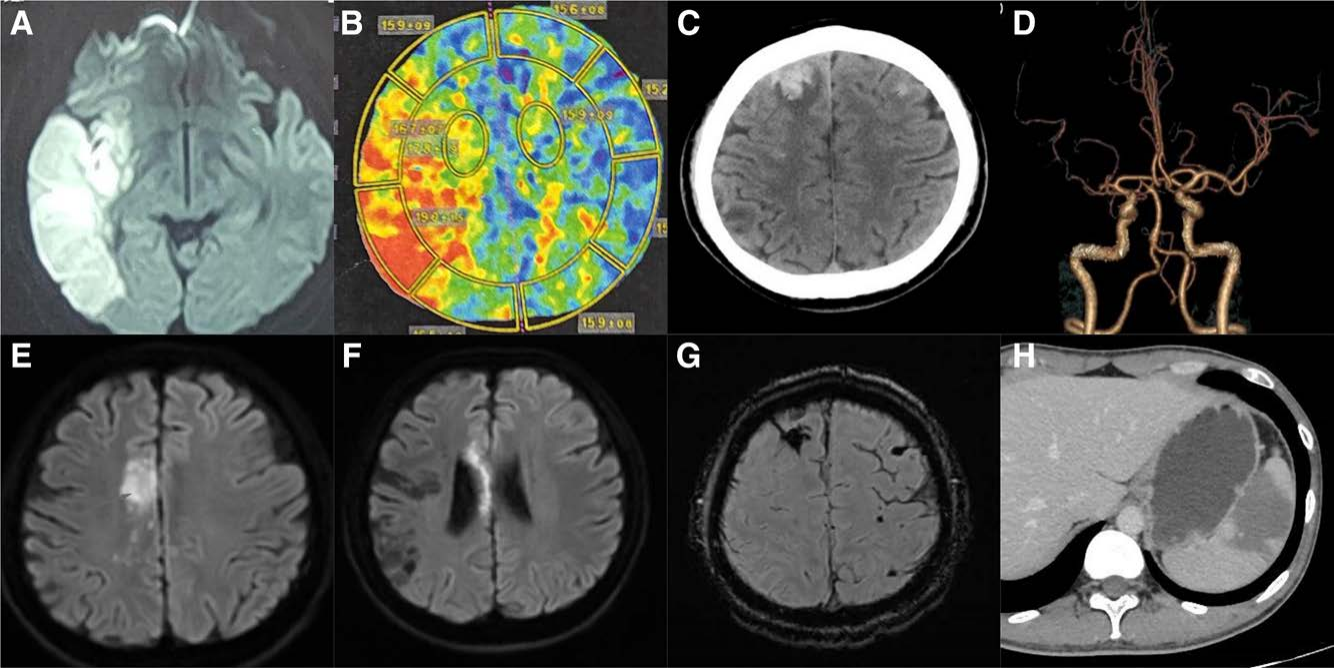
(A) Cranial MRI on June 22, 2021, showed: a large cerebral infarction in the right frontotemporal lobe and insula. (B) Cranial CTP on June 22, 2021, showed: severe stenosis of the proximal segment of M1 of the right middle cerebral artery nearly occluded, with sparse distal branching, ischemia, and hypoperfusion of the corresponding blood-supplying area, 62 mL of ischemic semidarked band, and large cerebral infarction in the right frontotemporal lobe. (C) Cranial CT on September 23, 2021, showed: patchy high-density shadows with clear margins were seen in bilateral frontal lobes, and low-density shadows were seen around them. (D) Cranial CTA on September 23, 2021, showed occlusion of the M1 segment of the right middle cerebral artery. (E) Cranial MRI on January 29, 2022, showed DWI sequences, and diffusely restricted signals were seen in the right frontal-parietal lobe. (F) Cranial MRI on January 29, 2022, showed DWI sequences, and a focally low signal was seen in the right corpus callosum. (G) Cranial SWI sequence on January 29, 2022, bilateral frontal-parietal lobe and part of the sulcus, right temporal lobe see low signal shadow. (H) Abdominal CT on February 12, 2022, showed splenic infarct foci. CT = computed tomography, CTA = computed tomography angiography, CTP = computed tomography perfusion, DWI = Diffusion-weighted imaging, MRI = magnetic resonance imaging, SWI = susceptibility weighted imaging.
